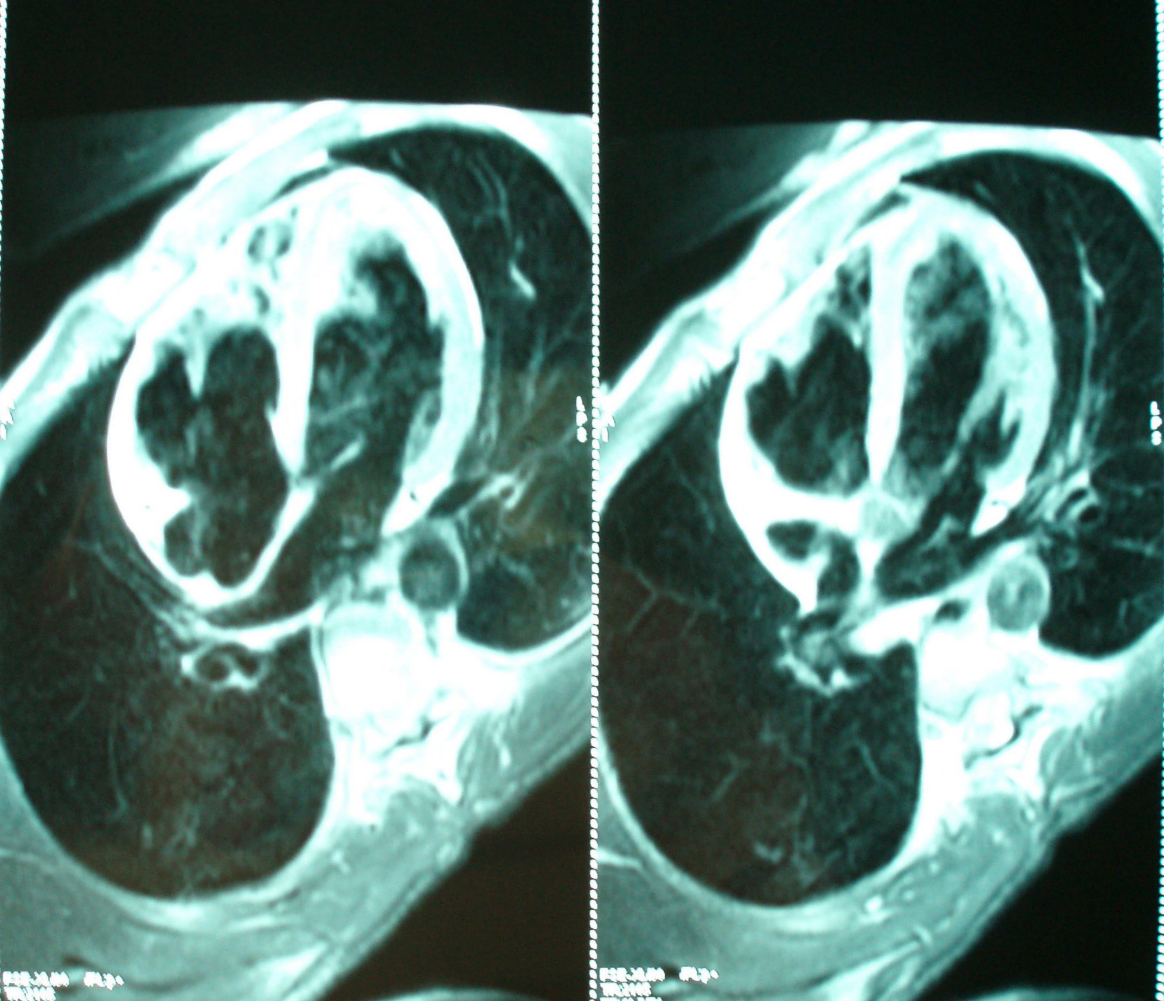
Four chamber view showing the trabeculations of the right ventricular wall by Magnetic resonance imaging.
